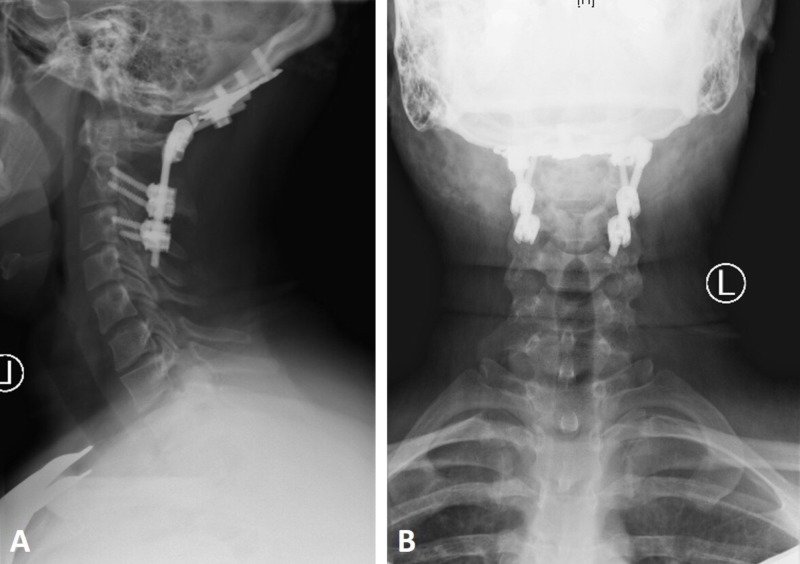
Occipitocervical fusion Lateral and anteroposterior cervical x-rays showing occipitocervical fusion from the occiput to C3 showing appropriate alignment at the craniovertebral junction (CVJ).
